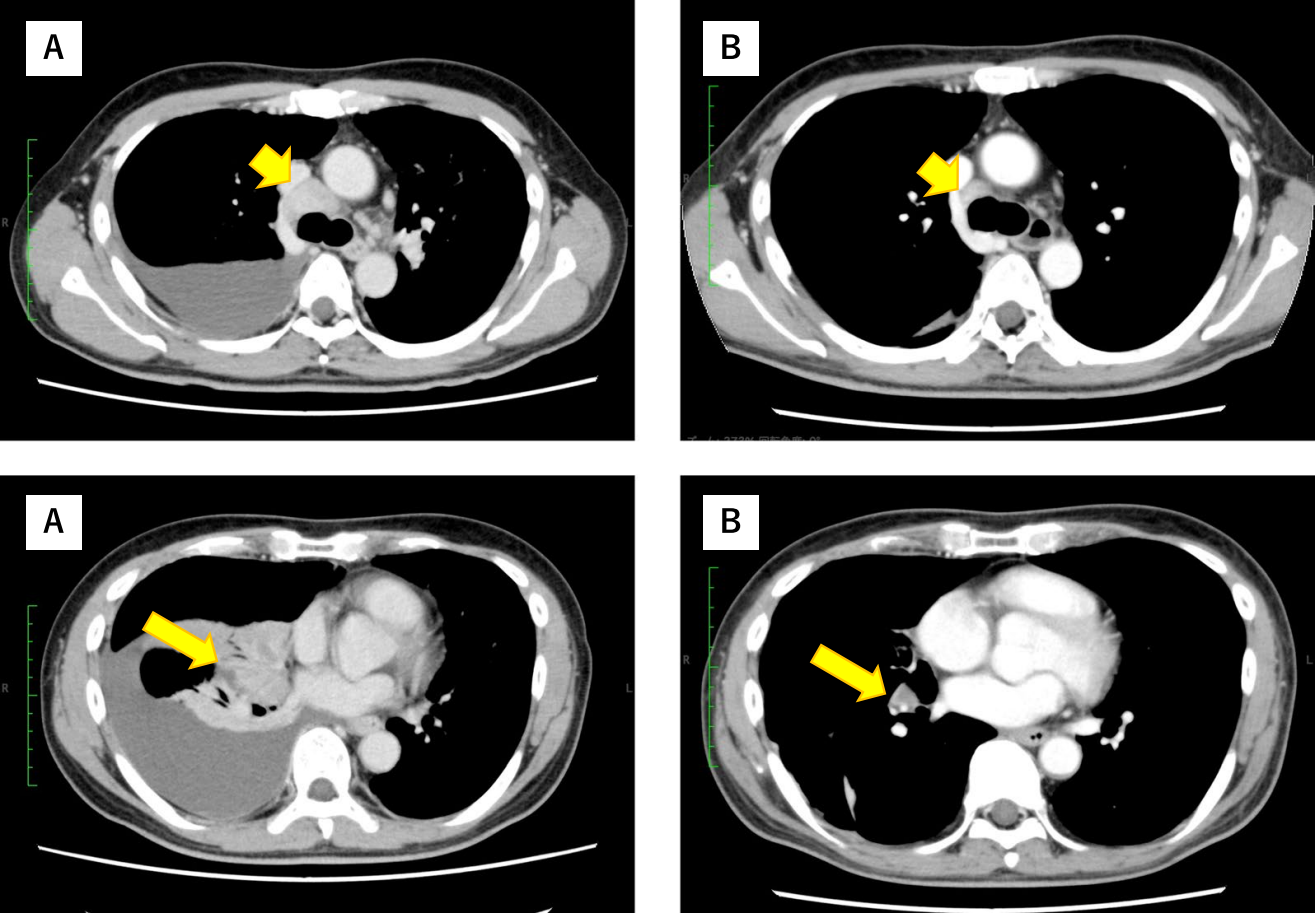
Chest computed tomography images before and after administering crizotinib. a Primary lung cancer in the right lower lobe, mediastinal lymph node metastasis, and right pleural effusion before treatment. b Significant tumor reduction in the primary tumor and (long arrow) mediastinal lymph node metastasis (short arrow). The right pleural effusion had disappeared after administering crizotinib
